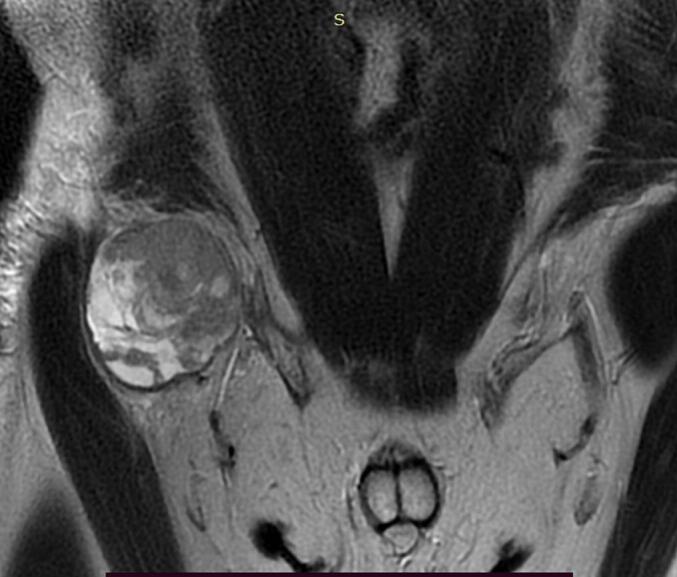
Pelvic MRI displaying a hyperintense mass on T1-weighted imaging developed in the right inguinal region behind the inguinal ligament and the inguinal fascia. It measures 40 mm in antero-posterior diameter, 60 mm in height, and 62 mm in transverse diameter.
